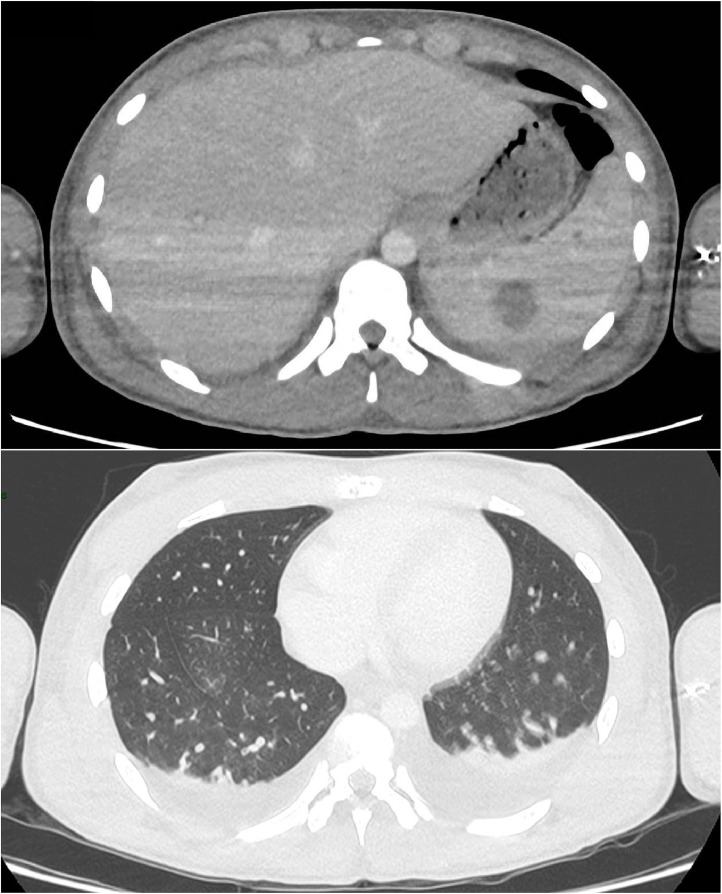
Hepatosplenic and pleuropulmonary lesions. CT scan performed at Day 10. Adenomegalia of the celiac region and hepatic hilum. Hepatomegaly with one lesion of the left liver. Multiple splenic lesions.
